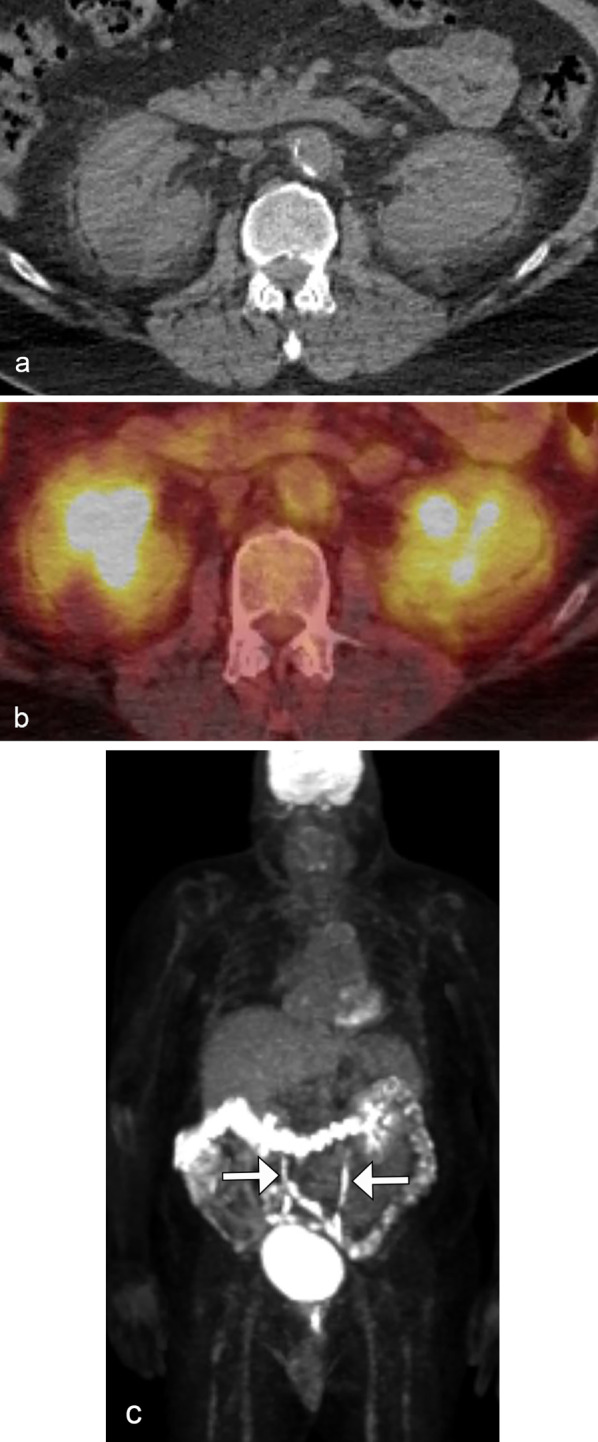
Erdheim–Chester disease.* Axial noncontrast CT (a) demonstrates bilateral perinephric soft tissue stranding/infiltration mimicking the perinephric infiltrative appearance of lymphoma. F18-FDG PET/CT images (b, c) demonstrate the low-grade metabolic activity associated with soft tissue stranding. Note the normal course of the bilateral ureters (arrows). The patient was also found to have thoracic periaortic soft tissue thickening and bilateral metadiaphyseal sclerosis which are additional features of Erdheim–Chester disease
